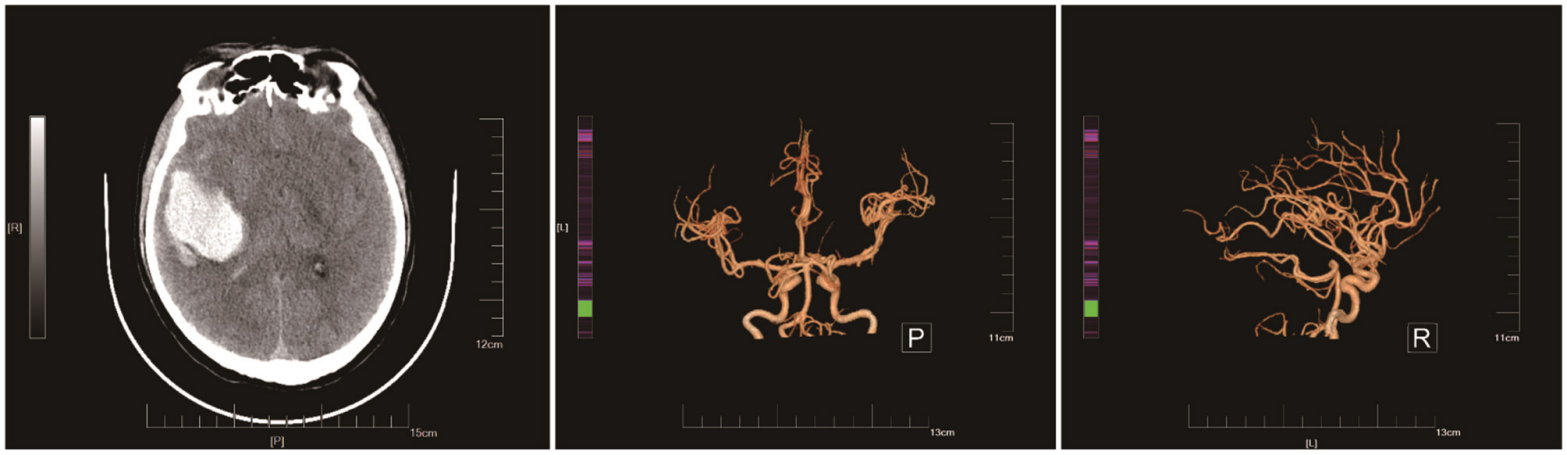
Preoperative brain CT images show a right temporal lobe cerebral hemorrhage and a diffuse subarachnoid hemorrhage, suggesting an Arteriovenous Malformation (AVM).
